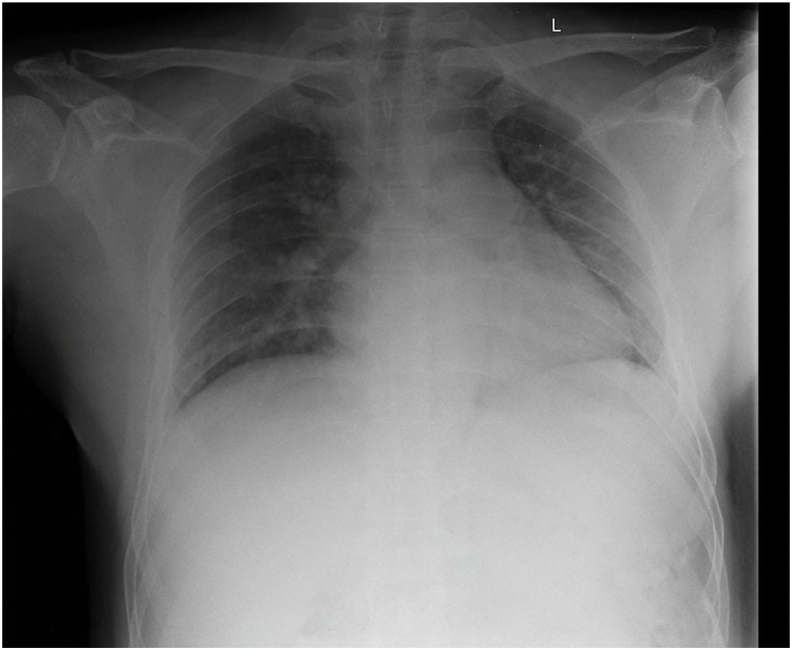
Chest X-ray revealed bilateral pneumonia.
